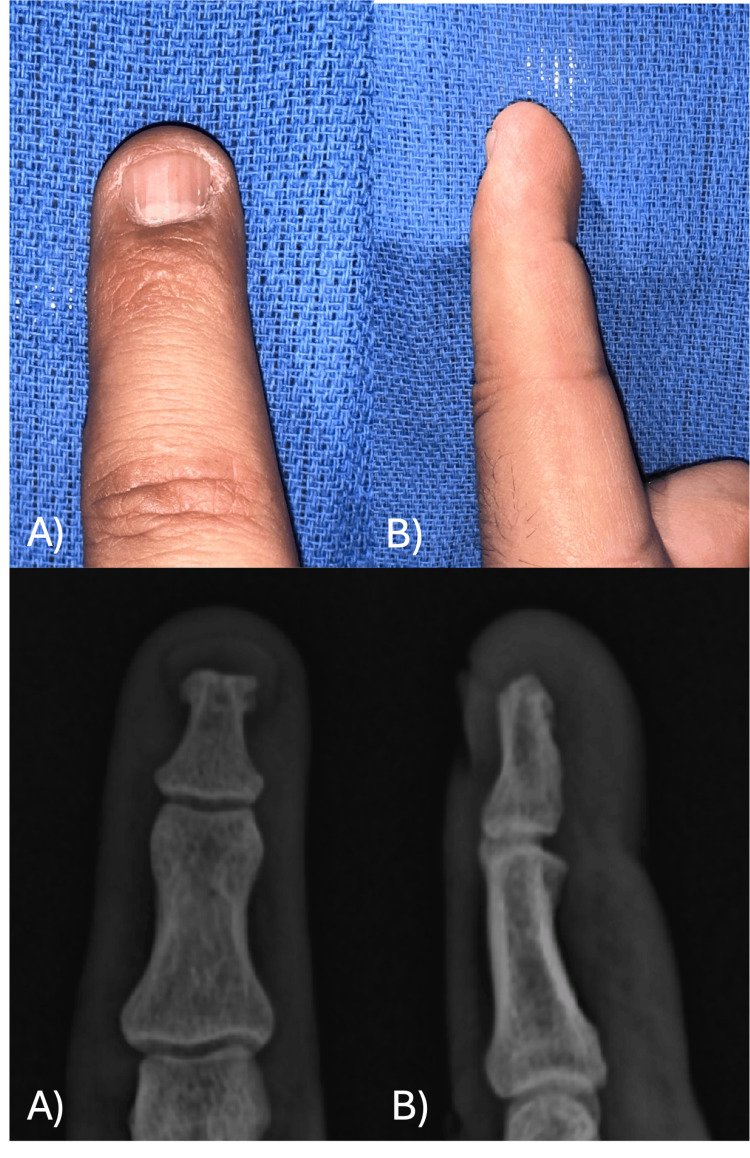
Two-year follow-up images in Case B Very satisfying functional and aesthetic results. (A) Posterior image of index finger. (B) Lateral image of index finger. (C) Posterior X-ray projection. (D) Lateral X-ray projection
